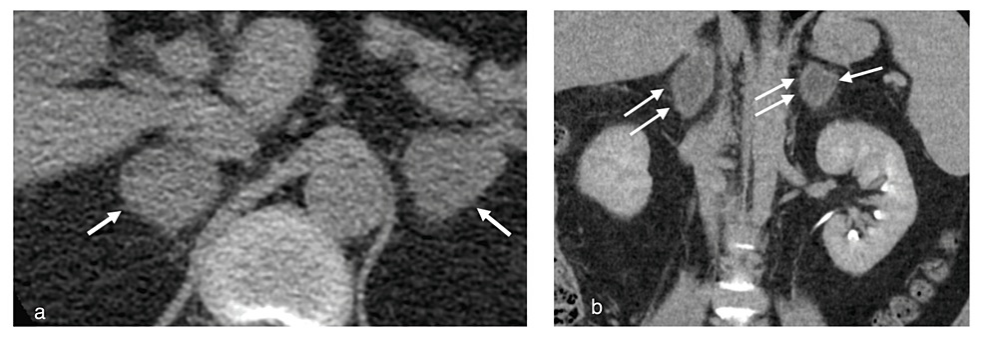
(a) Pre-contrast CT image showing bilateral adrenal masses (arrows). (b) Post-contrast images showing focal preservation of normal adrenal enhancement in the periphery (arrows)
